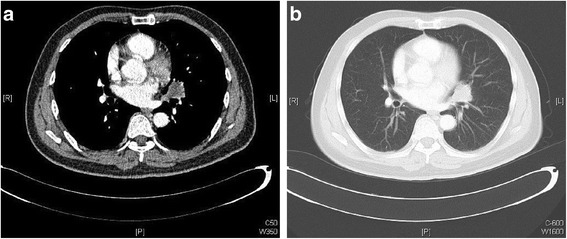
Lung metastases of case 1. Computed tomography scan of the lungs revealed multiple nodular lesions. a Mediastinal window; b pulmonary window
